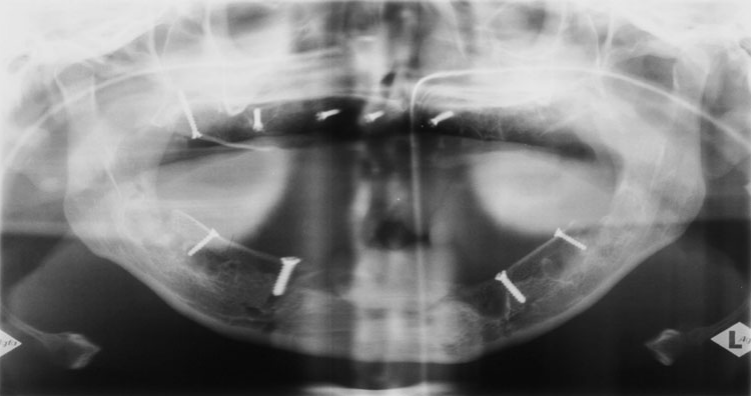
postoperative panoramic radiographs after tooth extraction and bone augmentation.
